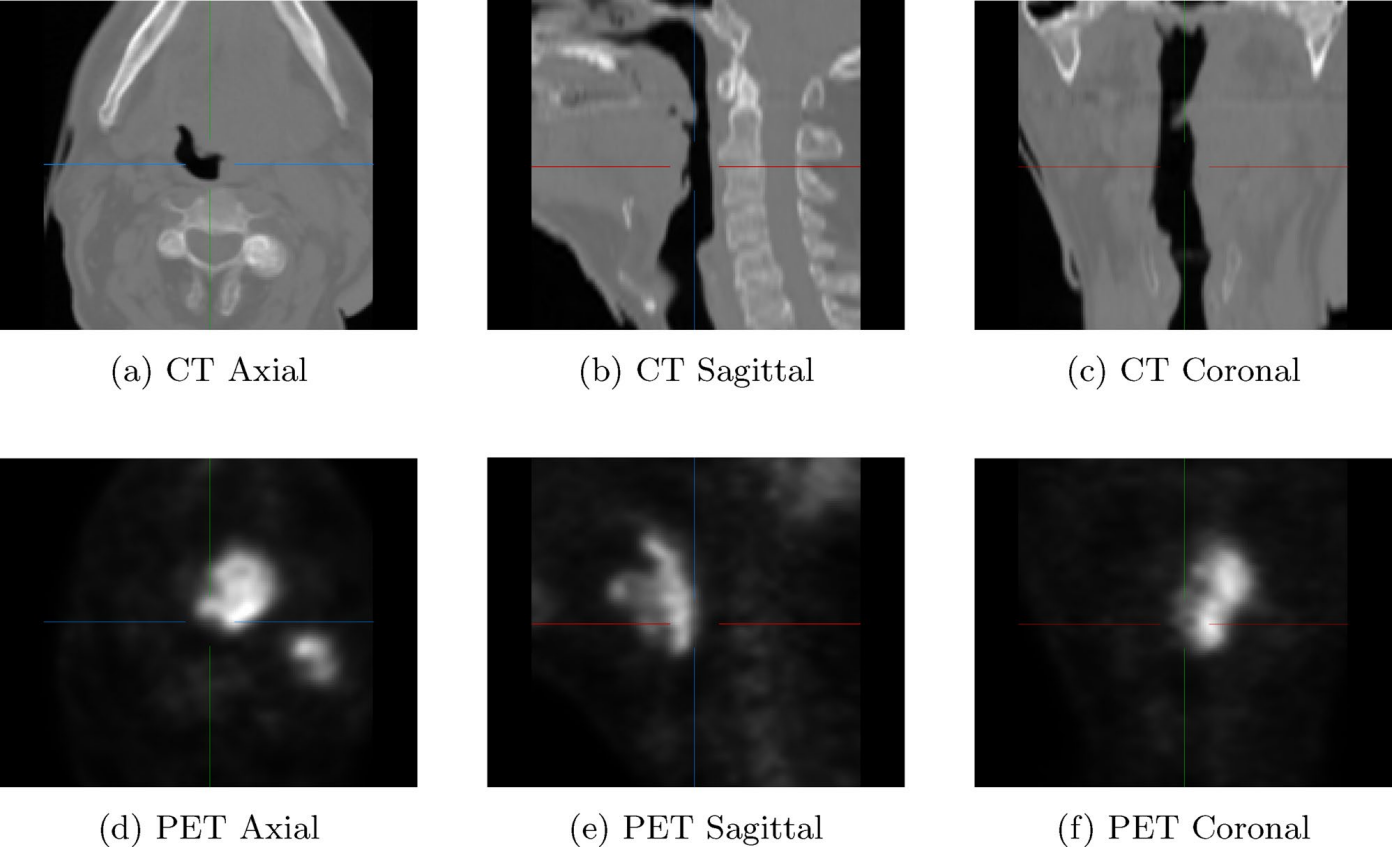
Axial, sagittal, and coronal views of the CT (top row) and PET (bottom row) images from the HECKTOR dataset. These images provide a comprehensive visualization of the anatomical and metabolic information, with the CT scans highlighting the structural details and the PET scans showing metabolic activity within the same regions
